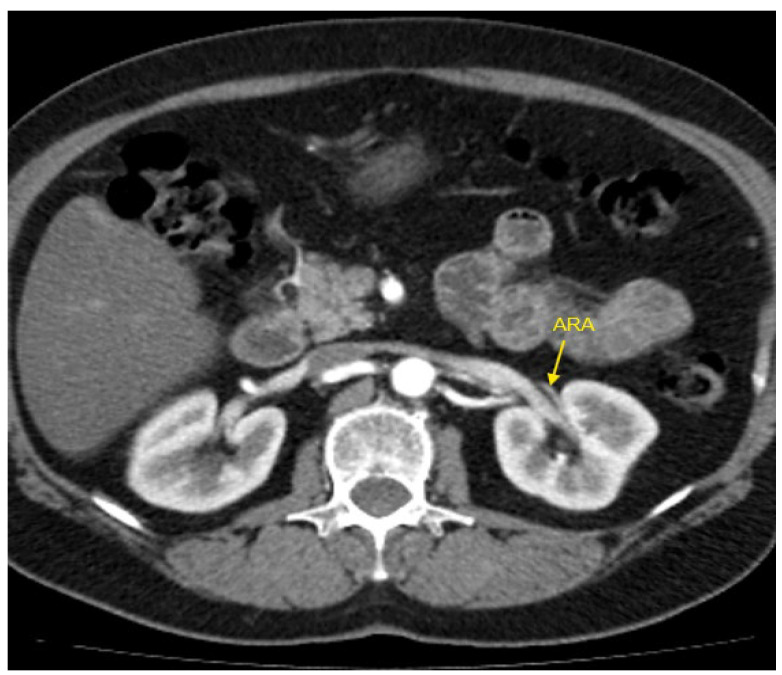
Contrast-enhanced CT imaging of a 47-year-old female patient with refractory hypertension revealed an accessory renal artery in the left kidney, shown by the yellow arrow. ARA—accessory renal artery; CT—computed tomography.
